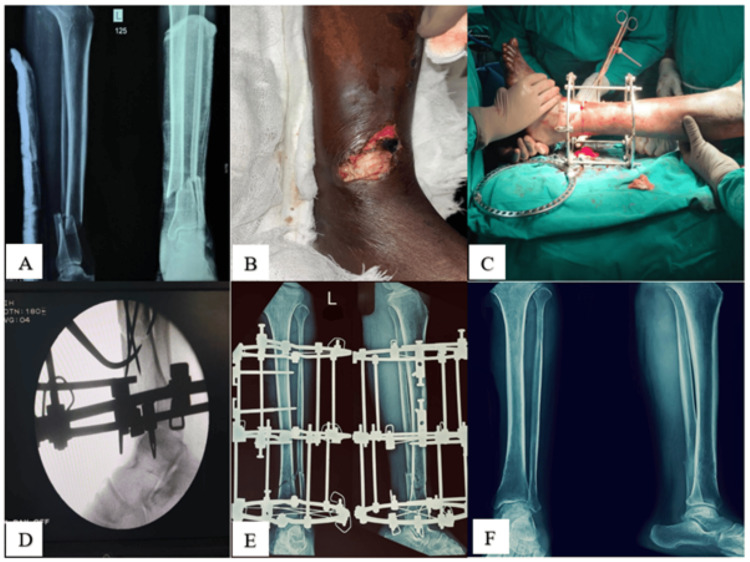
Representative Case of Gustilo-Anderson Grade IIIB Open Tibial Fracture Managed With Ilizarov External Fixator (A) Preoperative radiograph showing tibial fracture; (B) preoperative clinical photograph demonstrating soft tissue defect; (C) intraoperative image during application of Ilizarov external fixator; (D) intraoperative C-arm image confirming fracture alignment; (E) postoperative radiograph showing fixator in situ; and (F) follow-up radiograph demonstrating fracture union.
